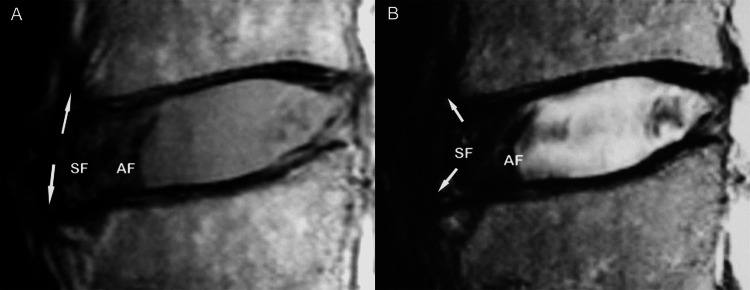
Sharpey fibers on MRI. Sagittal T1-weighted (A) and T2-weighted (B) MR images display the site of origin (thin arrows) of strong Sharpey fibers (SF) at the periphery of the annulus fibrosus (AF), in the region of the ring apophysis.  This is the exact site of anchorage of the annulus to the anterior vertebral surface.
